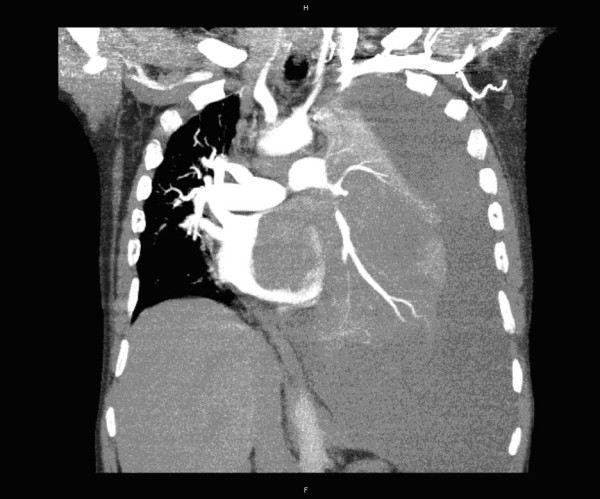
Computed tomography shows a large mass in the left pleural space penetrating the left pulmonary veins and protruding to the left atrium and through the mitral valve.
